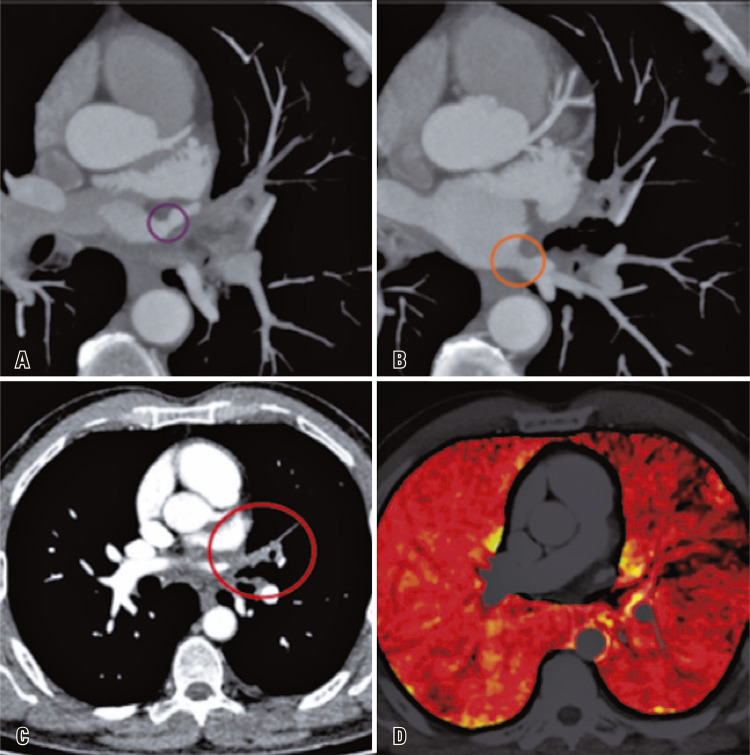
Computed tomography pulmonary angiogram demonstrates a focal reduction in the caliber of both the ostia of the left upper pulmonary vein (purple circle in image A) and left lower pulmonary vein (orange circle in image B); occlusion of pulmonary venous branches upstream of the stenotic point is observed (red circle in image C) along with corresponding segmental pulmonary hypoperfusion within the territory drained by the stenosed veins is evident in the iodine map images (image D)
